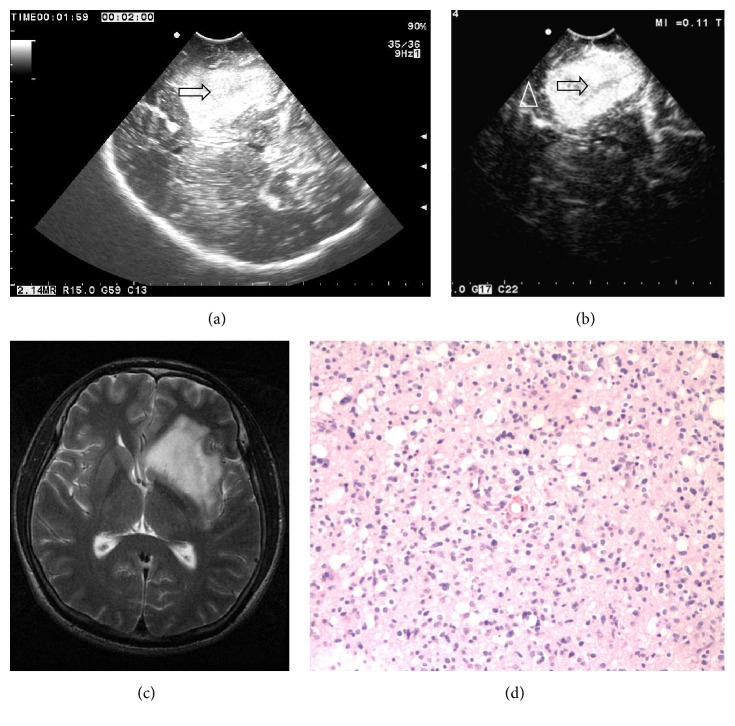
(a) Ultrasound image shows that the low grade glioma (II level) is partially hyperechoic, the boundary is not clear, internal echo is nonuniform, and edema is not obvious (arrow showed tumor). (b) CEUS shows that the echo of the glioma is enhanced significantly, the boundary is clear, and normal brain tissue is enhanced lower than the glioma (arrows show tumor; triangle shows normal brain tissue). (c) MRI T2W image shows that the glioma is hyperintense, irregular, and the boundary is not clear. (d) The pathological image of the same patient shows that the number of capillary vessels is less (HE ×100).
